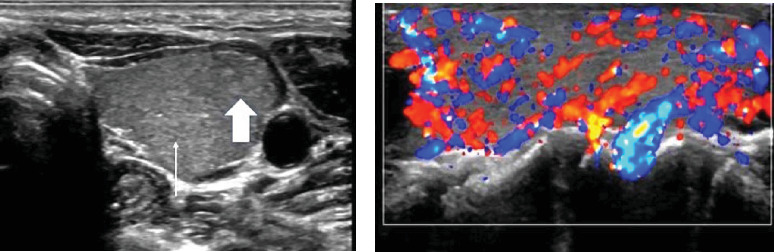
B-mode (a) and Duplex (b) ultrasonographic images of the thyroid gland of the 23-year-old patient, showing coarsened parenchyma echotexture (thin arrow) and multiple hyperechoic nodules consistent with pseudonodular appearance (thick arrow) on B-mode, while on Doppler ultrasound, it shows hypervascularity. These appearances are highly specific for Hashimoto thyroiditis.
